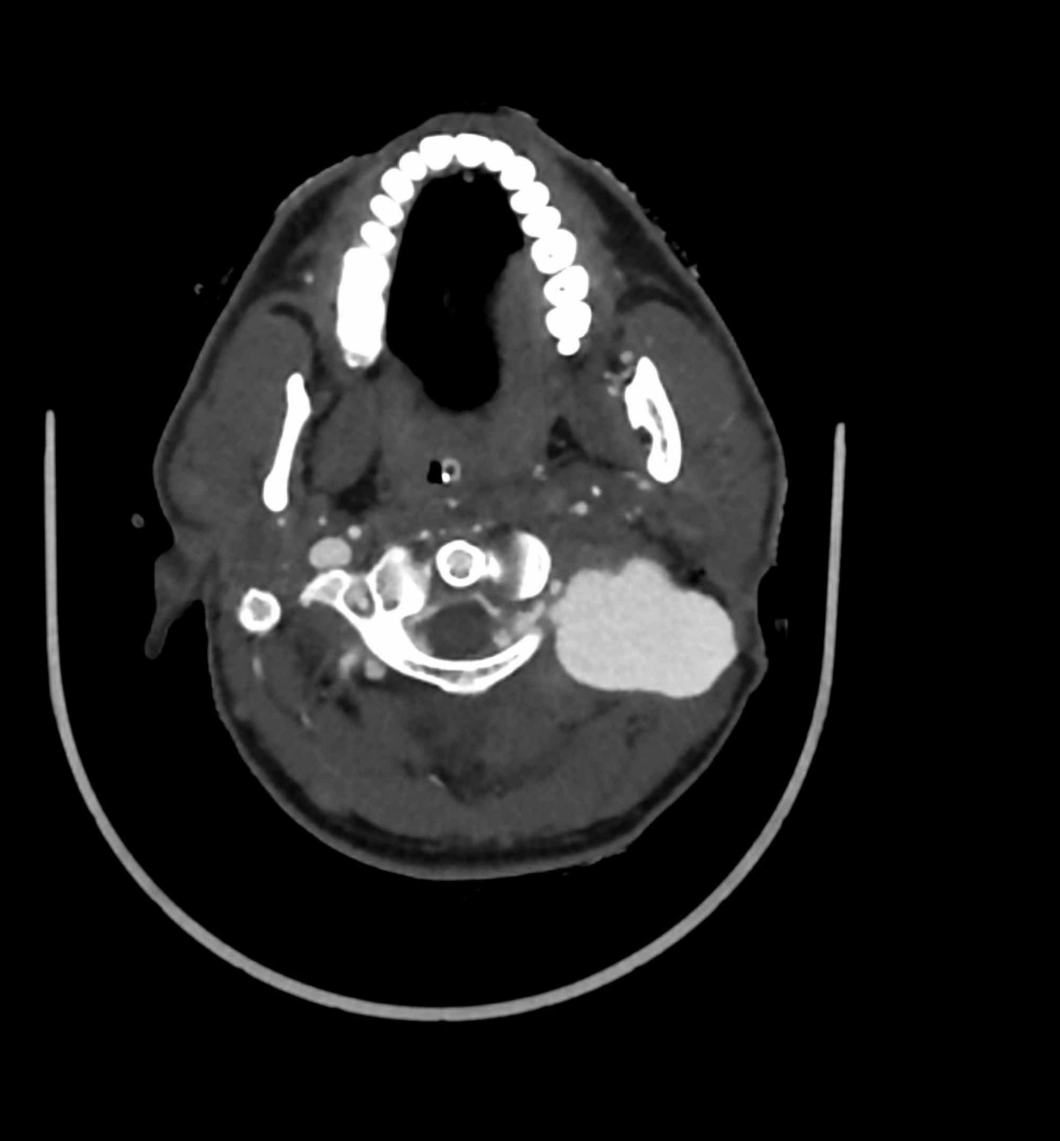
CT angiogram shows a pseudoaneurysm arising from the V2 segment of the left vertebral artery. The epidural venous plexus appears engorged which is suggestive of a vertebral arteriovenous fistula.
